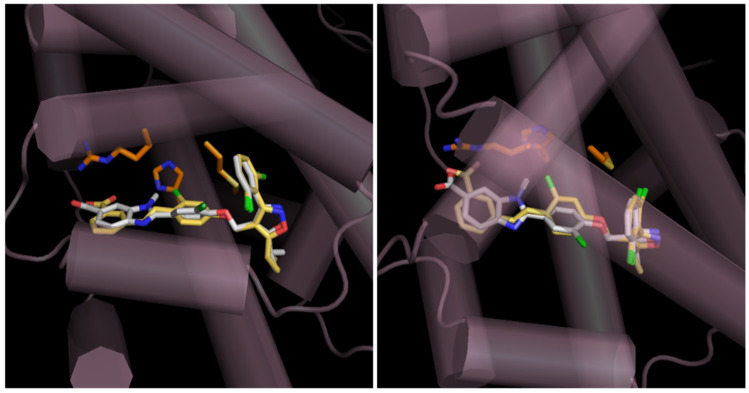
Modeling of FXR complexed with GW4064 (1) and 23. A complex model of human FXRα-LBD monomer (PDB ID: 3DCT, purple cylinders and orange sticks) with GW4064 (1) (X-ray; yellow sticks) and 23 (model; white sticks) was built using AutoDock Vina1.1.2. Left panel: view from the side. Right panel: view from above. Reprinted with permission from Ref. [53].
